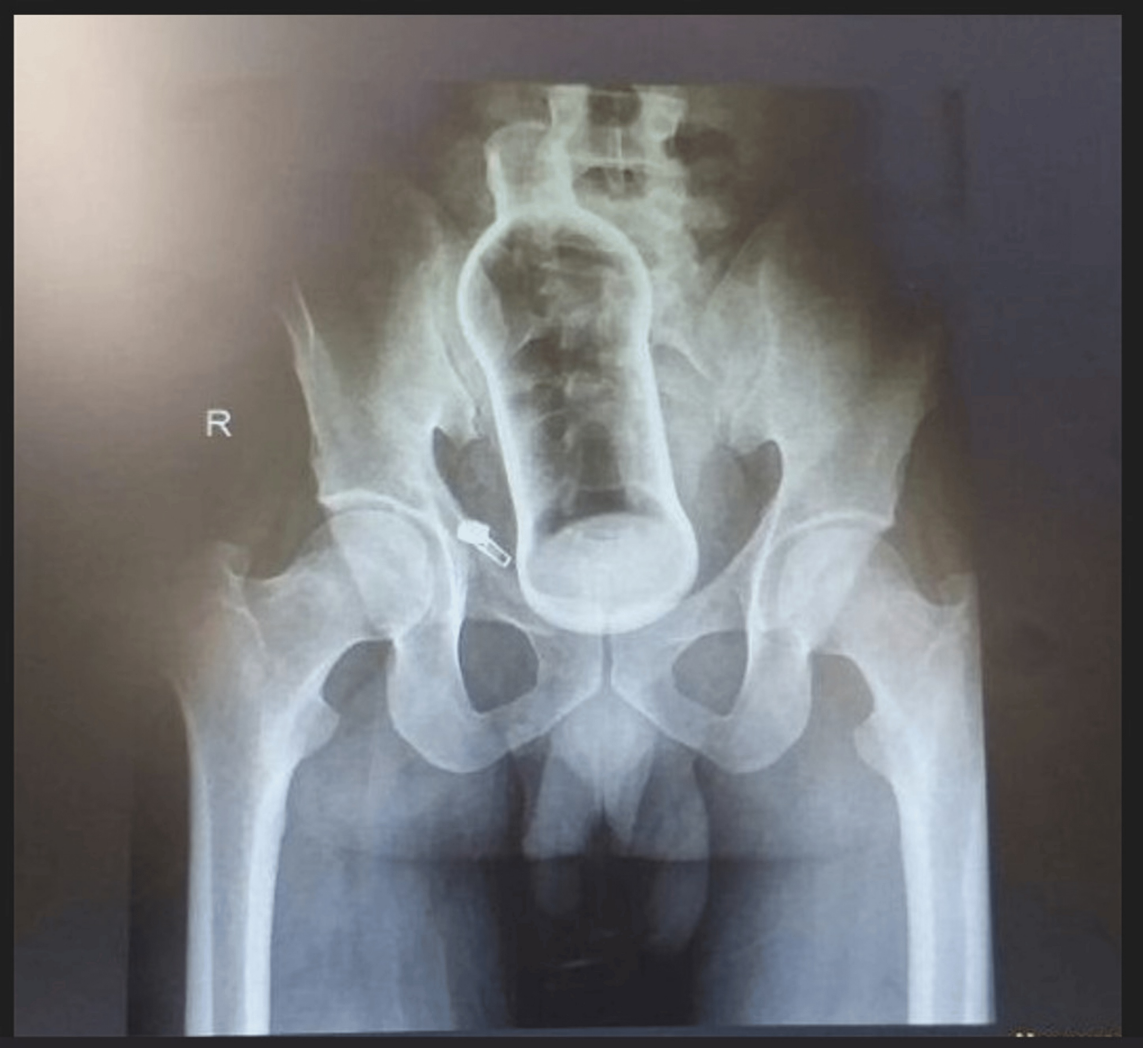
Pelvic X-ray anteroposterior view revealing a foreign body
